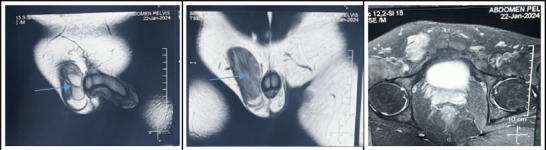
MRI image, arrow shows both testis in right inguino scrotal sac (A); Right inguinal defect through which omentum is herniating (B); rudimentary uterus and fallopian tubes lying behind the bladder (C).
